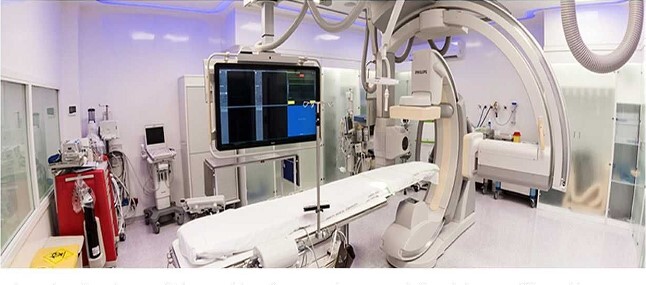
The angiography room with the state-of-the-art floor-mounted X-ray system (Allura Clarity FD20, Philips Healthcare, Best, Netherlands) installed in 2016 is shown.
